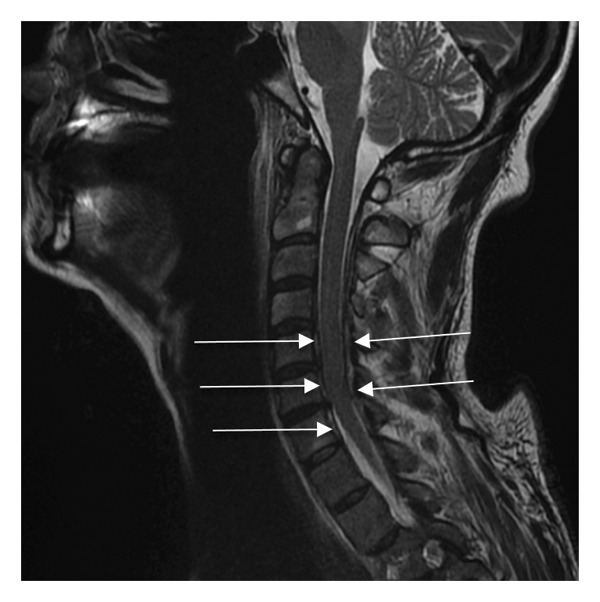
Sagittal MRI. Circumferential epiduritis from C3 to C7.
